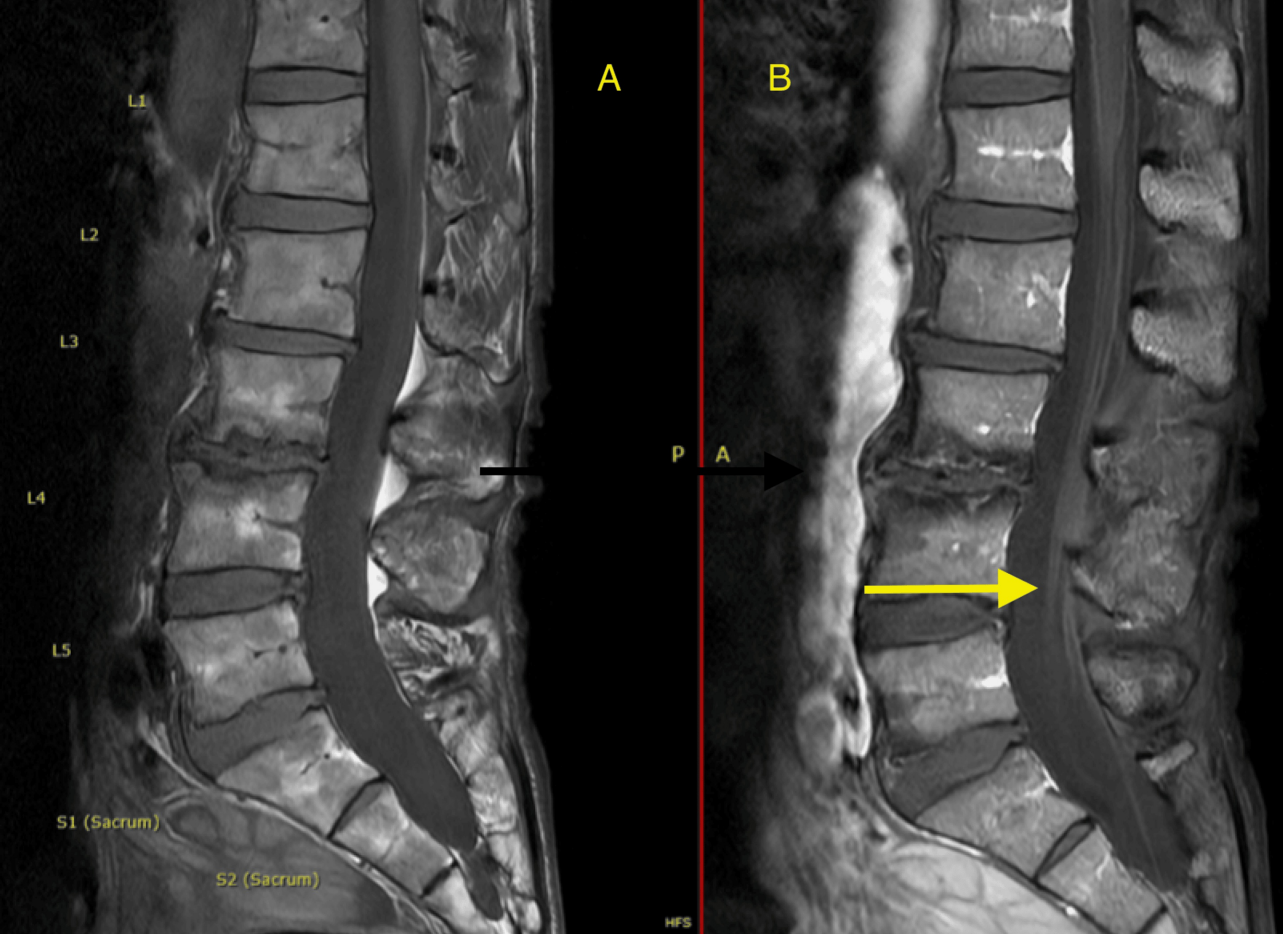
MRI lumbar spine with and without contrast. (A) Precontrast and (B) postcontrast image with evidence of cauda equina enhancement (yellow arrow).
